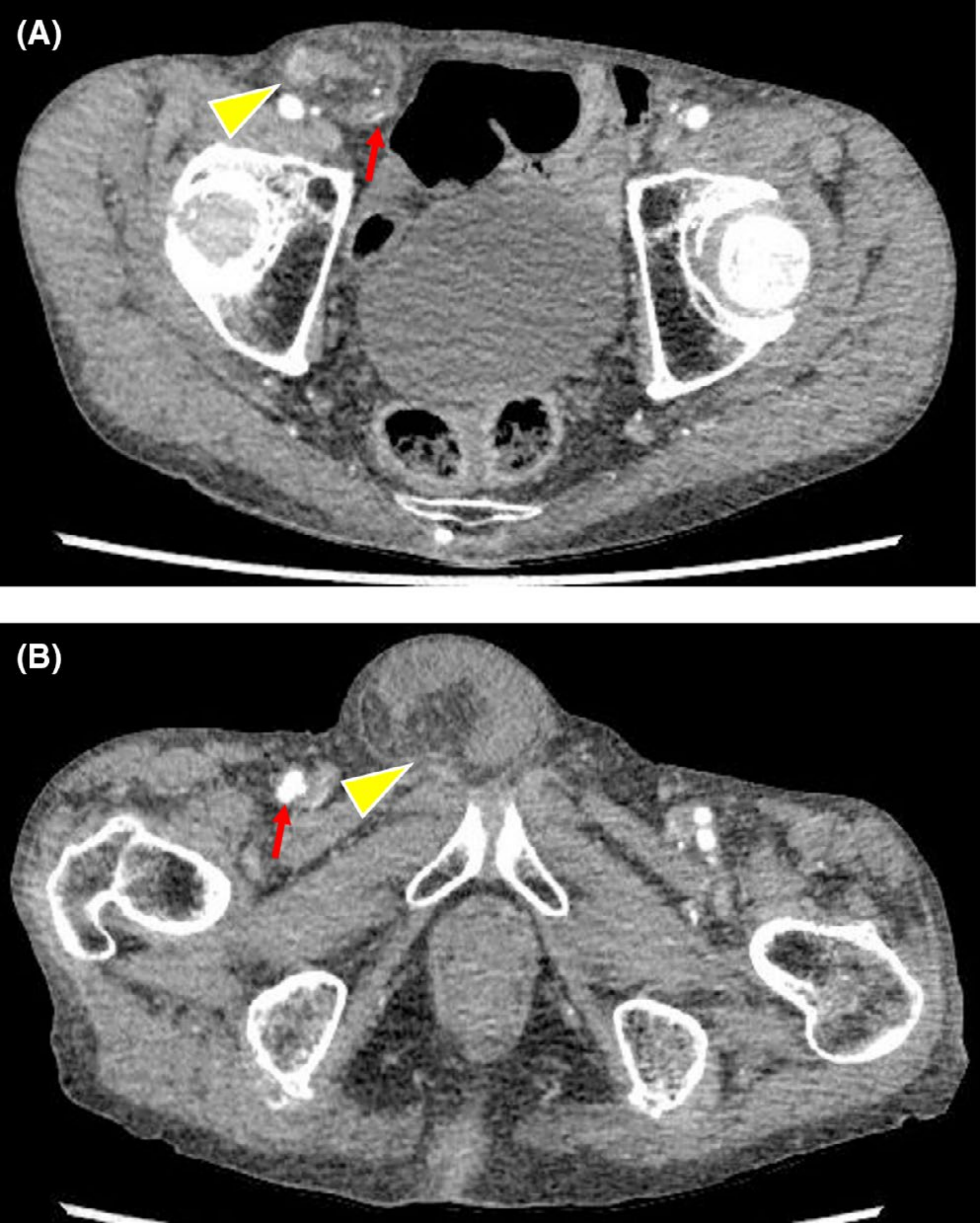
Abdominal contrast‐enhanced computed tomography findings. External inguinal hernia in the right groin (△). No obvious hernias were found in the left side. (→) indicates the lower abdominal wall artery. No obvious femoral hernia was found in the right groin. No evidence in the groin. (△) indicates inguinal hernia and (→) indicates the lower abdominal wall artery
